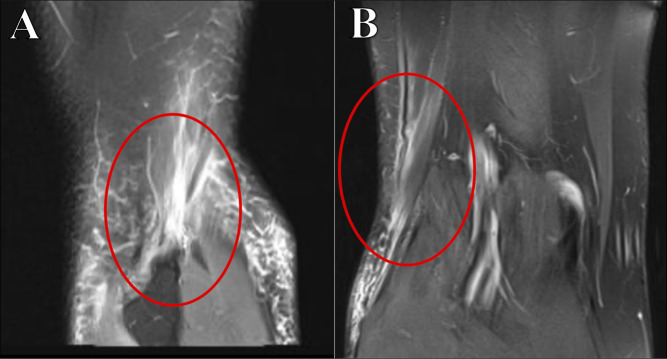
Sagittal T2 PDW MRI slice (A) demonstrating a distal avulsion of the biceps femoris tendon with the fibular head and retracted tendon end circled in red. There is fluid/edema between the visible tendon end and the fibular head with some tendon stump remaining on the fibular head. Coronal T2 PDW MRI slice (B) demonstrating retracted biceps femoris tendon. Note the nonlinear appearance or buckling of the tendon farther proximal to the ruptured end.
